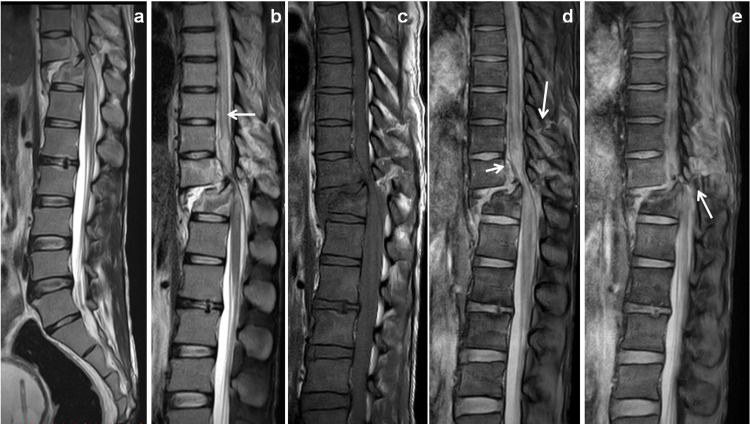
Displaced vertebral fracture with near-total cord dissection. Day 2 MRI scan of 28-year-old male patient with history of RTA. Sagittal T2W, STIR and T1W images (a, b & c) showed displaced fractures of D12 vertebra with teared longitudinal ligaments, ligamentum flavum and long segmental dorsal cord edema extends up to D7 vertebral level (← arrow). Sagittal mFFE images (d & e) showed ventral epidural hematoma (→ arrow). Hyperintense fractures noted in the D9, D10, D11 and D12 vertebrae (↓ arrow). Near-total cord transection was noted with teared posterior ligamentous complex affecting ligamentum flavum, Interspinous and supraspinous ligaments (↑ arrow). STIR: short tau inversion recovery; mFFE: multiecho fast field echo; RTA: road traffic accident.
